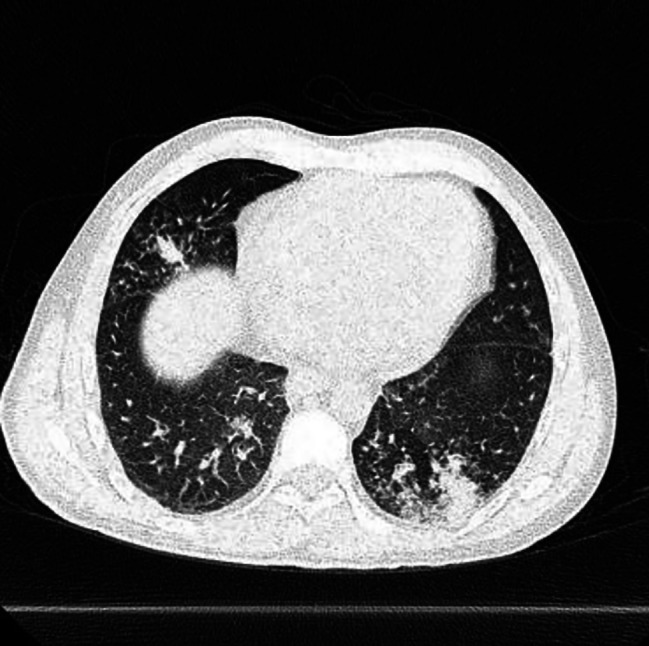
Axial CT image demonstrates nodules and consolidative opacities in right middle lobe and posterior left lung base
